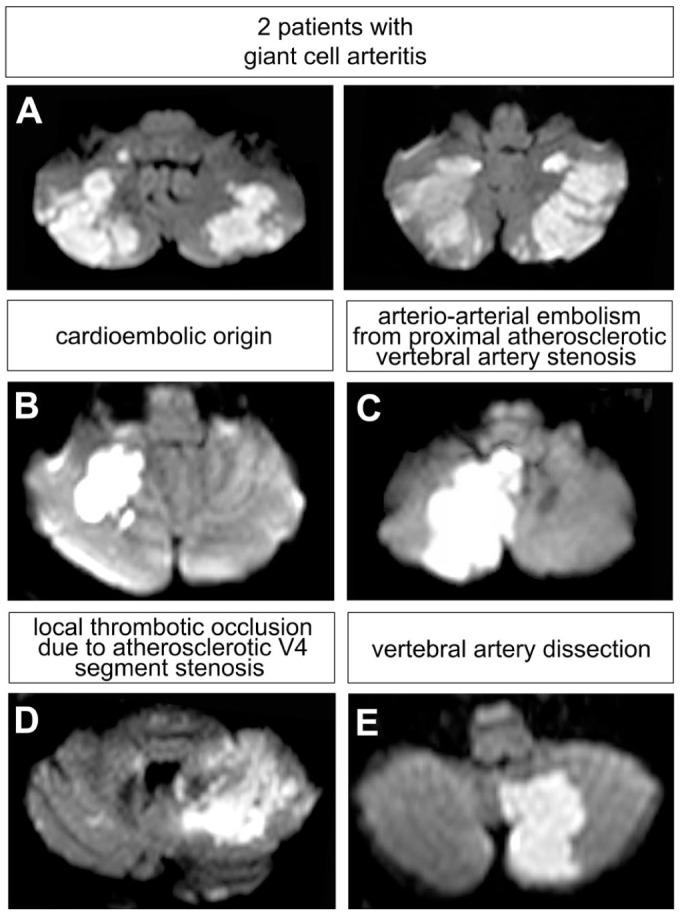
Axial diffusion-weighted MRI (DWI) scans of cerebellar infarctions of different etiologies. (a) Infarct distribution in patients with GCA and intracranial involvement (*n* = 2). (b) Representative infarct distribution in a patient with cardioembolic origin. (c) Representative infarct distribution in a patient with arterio-arterial embolism due to proximal atherosclerotic vertebral artery stenosis. (d) Representative infarct distribution in a patient with local atherosclerotic V4 stenosis. (e) Representative infarct distribution in a patient with vertebral artery dissection. GCA, giant cell arteritis.
